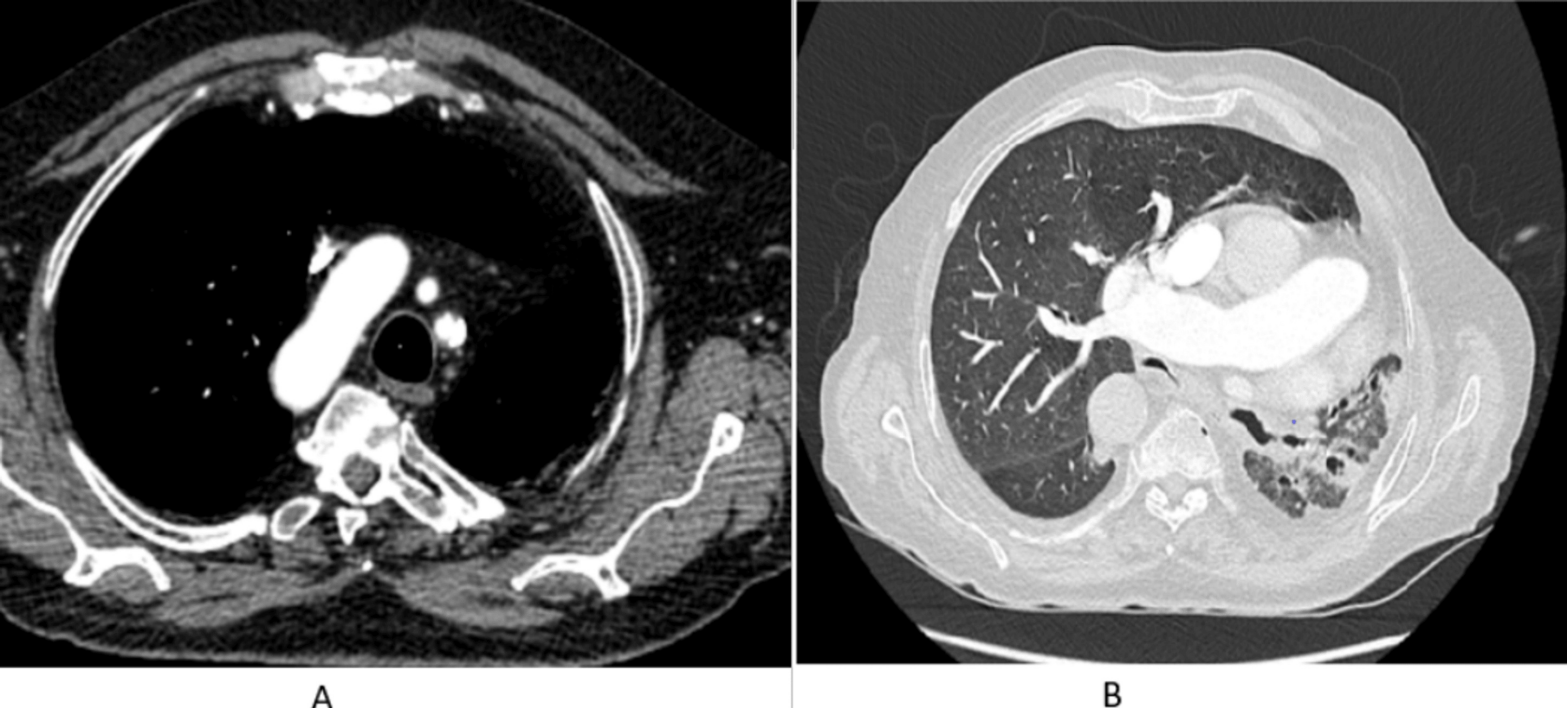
(A) Mediastinal window of the thoracic angioscan demonstrating the absence of the left pulmonary artery in conjunction with a prominent large-vessel malformation involving the right thoracic aorta. (B) Chest CT parenchymal window demonstrating hypoplasia of the left lung and left inferior lobar varicose bronchiectasis with an infectious appearance CT: computed tomography
